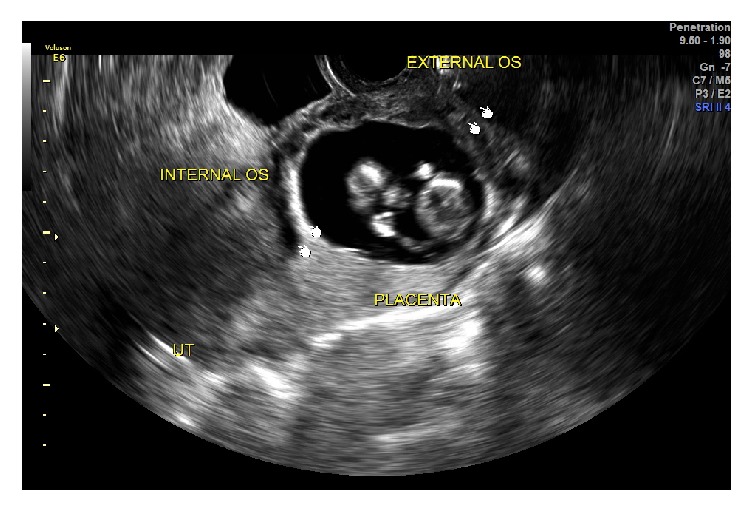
Transvaginal ultrasound: ultrasound imaging of viable cervical ectopic pregnancy. The fetus and placenta appear to be inferior or distal to the internal cervical os. The external os had about 1 cm of functional length.
